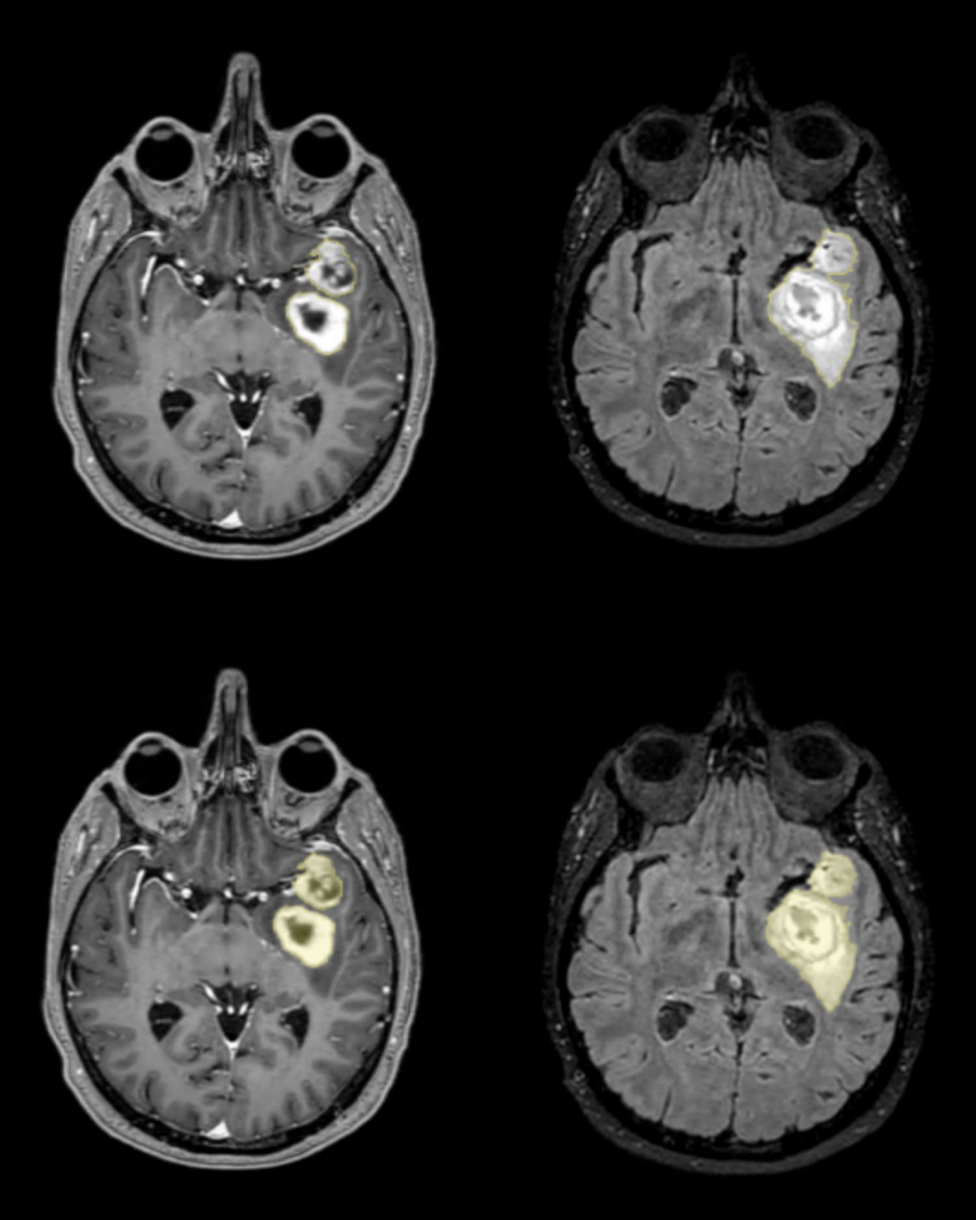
The outlines (top) and color overlap (bottom) of a GBM on a T1Gd slice are shown to the left. The images to the right are corresponding outline (top) and color overlap (bottom) of the peritumoral edema from the same tumor on a FLAIR weighted MRI slice. The delineations were performed with the semi-automatic software “3DSlicer” T1Gd = T1-weighted, Gadolinium contrast enhanced MRI. FLAIR = Fluid Attenuated Inversion Recovery sequenced MRI
